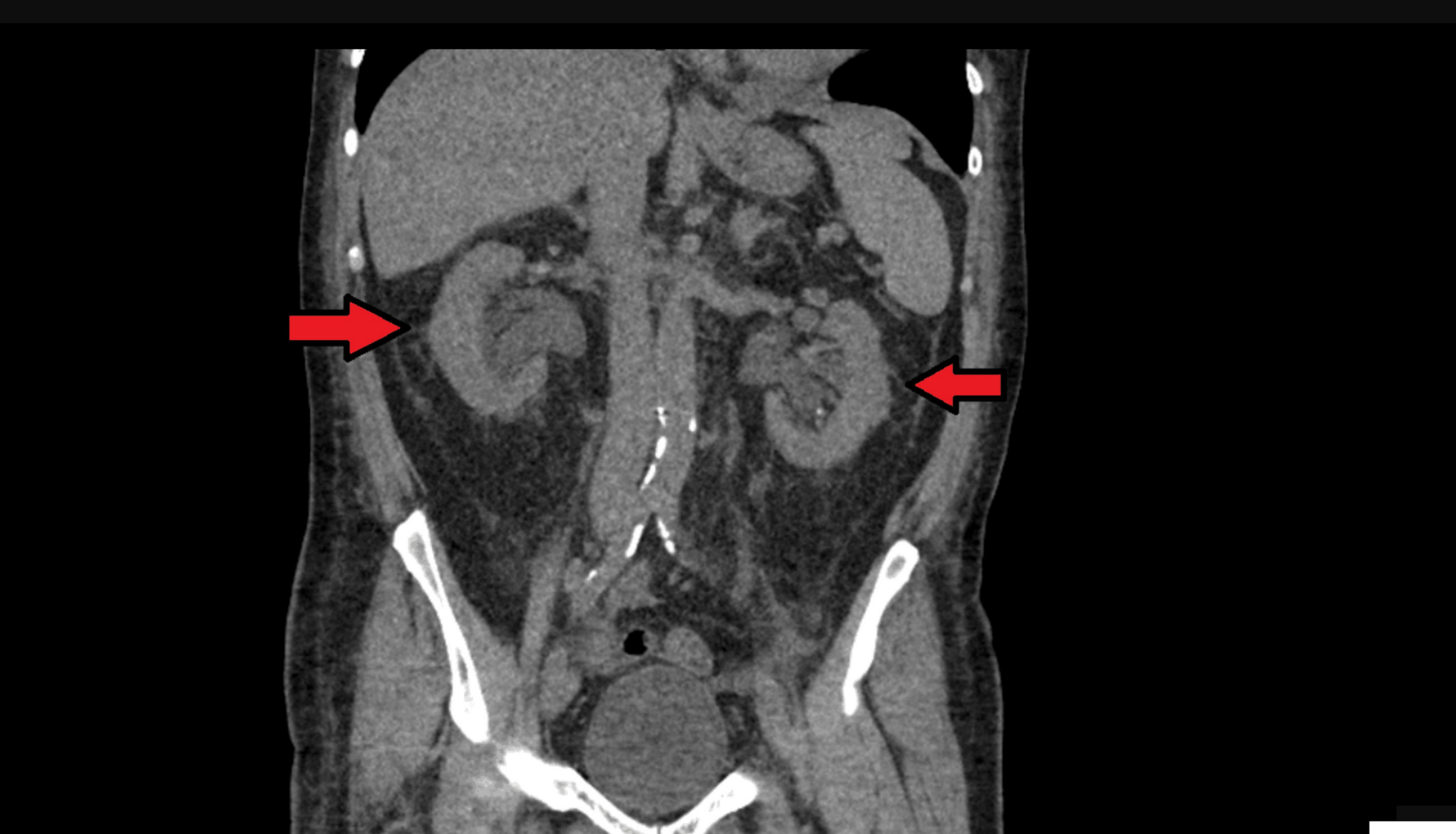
CT renal Bilateral arrowheads show evidence of severe hydronephrosis with moderate hydroureter bilaterally, without obvious cause. The kidneys are normal in size with severe hydronephrosis.
